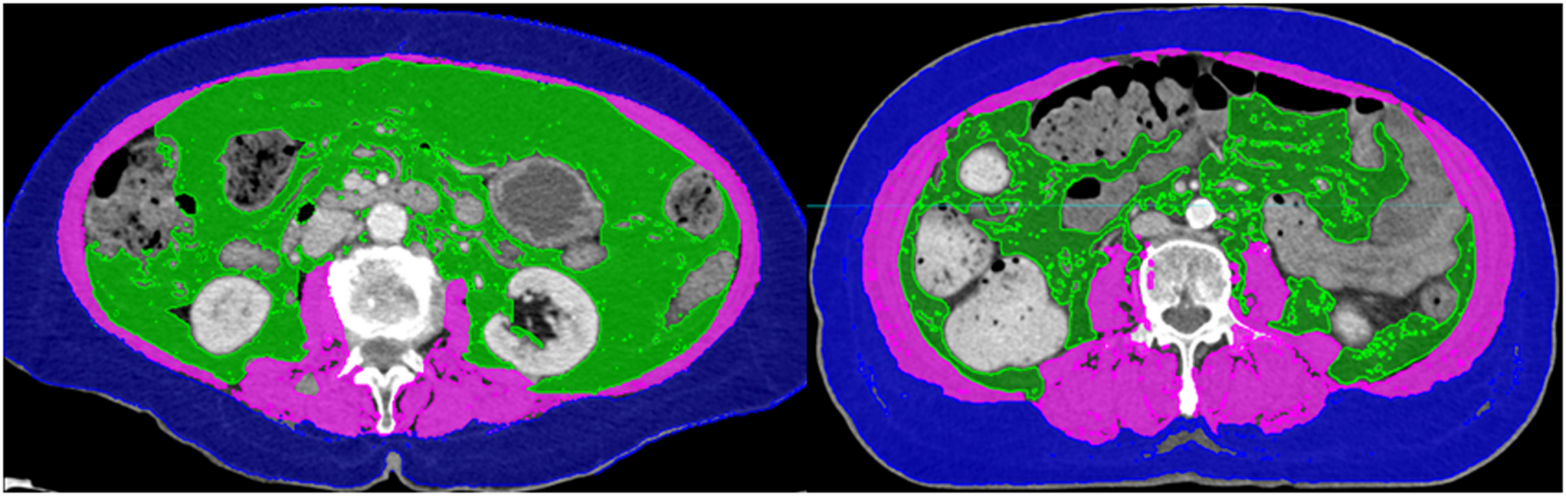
Computed tomography images of patients with (Left) and without sarcopenia (Right). The two patients had similar body mass indices.
